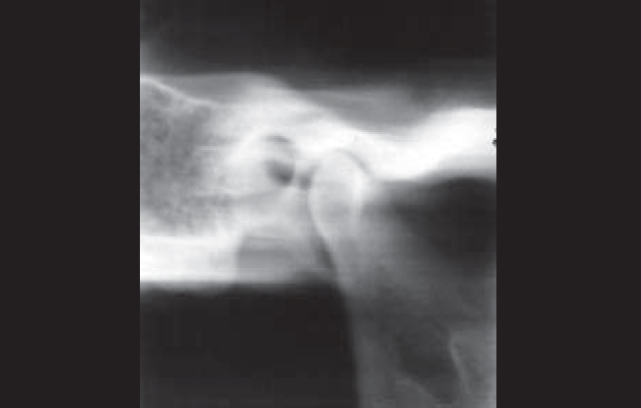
Corrected lateral CTs can - TMJ CT scan sections - section A without contrast material.
